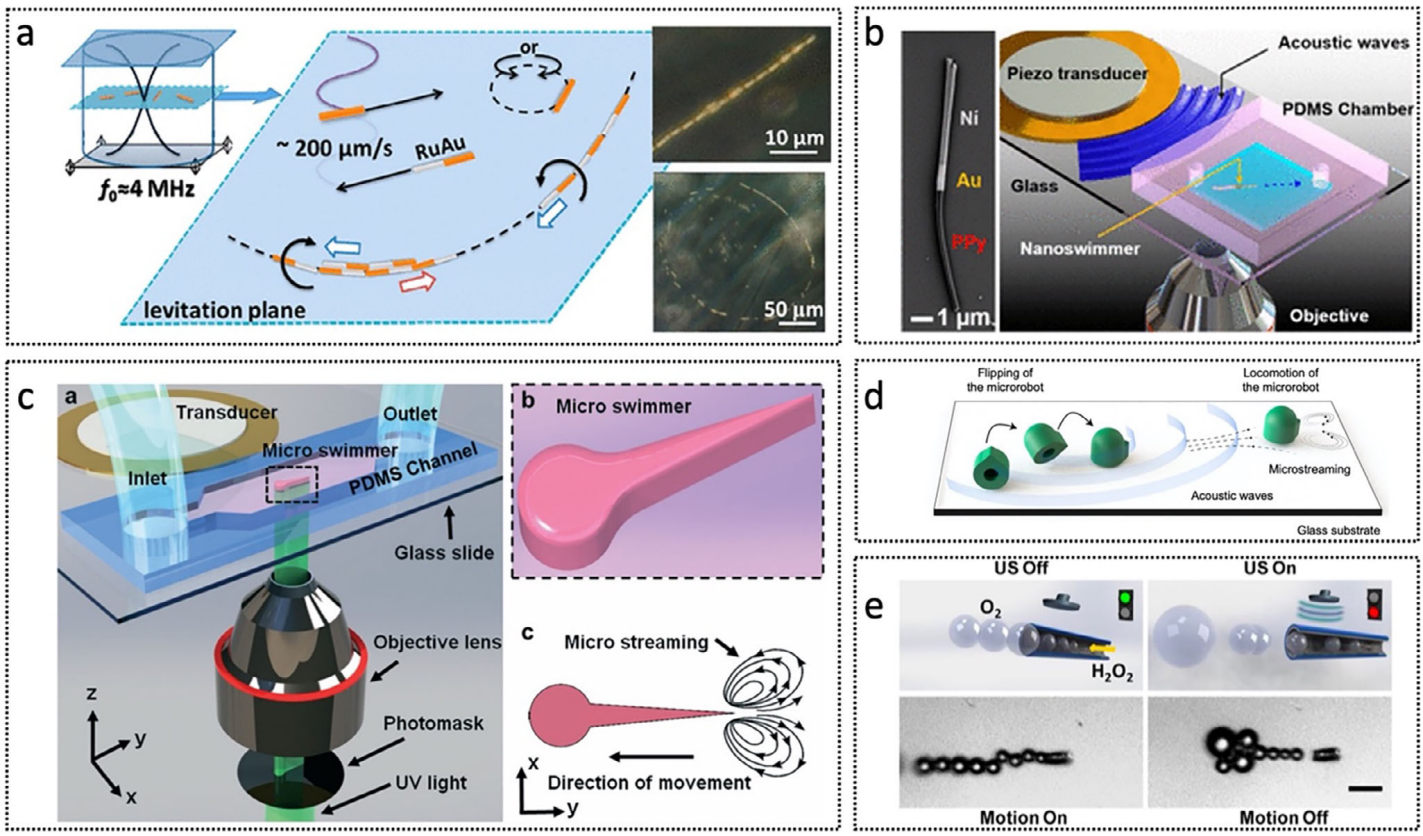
Acoustic‐driven micro/nanorobots. a) Acoustic metallic microrods aligning and self‐assembling into dynamic spinning chains with characteristic patterns, including ring and streak patterns. Reproduced with permission from ref. [75] Copyright, 2012. American Chemical Society. b) Nanoswimmer with a rigid bimetallic head and a flexible tail propelled by acoustically activated flagella. Reproduced with permission from ref. [78] Copyright, 2016. American Chemical Society. c) Fabrication and acoustic actuation of microswimmers. Reproduced with permission from ref. [79] Copyright, 2016. Royal Society of Chemistry. d) Acoustically driven bullet‐shaped microrobot. Reproduced with permission from ref. [81] Copyright, 2020. National Academy of Sciences. e) Ultrasound‐modulated bubble propulsion of chemically powered microengines. Scale bar, 20 µm. Reproduced with permission from ref. [82] Copyright, 2014. American Chemical Society.
